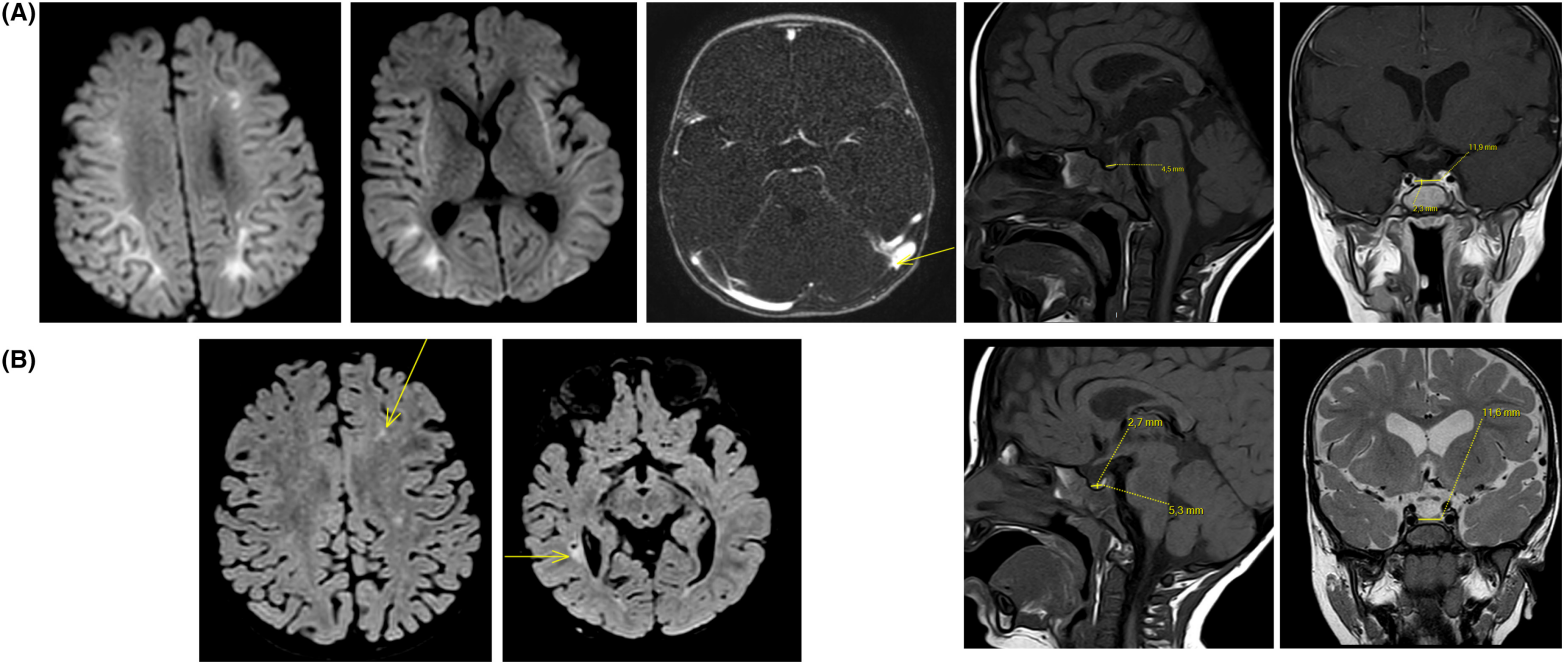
Magnetic resonance imaging performed on Day 2 and at 3 months. (A) MRI performed on Day 2, extensive thrombophlebitis of the superior sagittal sinus and of several cortical veins in the vertex, the torcular, the left transverse sinus and presence of small thrombi in the right transverse sinus. Significant bilateral and symmetrical signal abnormalities of the fronto‐parieto‐temporo‐occipital subcortical white matter and of the external capsules were also visible in diffusion restriction, which seemed rather to be related to metabolic disorders. (B) MRI at 3 months, partial permeabilization of the superior sagittal sinus with persistence of a heterogeneous aspect of the sinus, seat of some linear images probably corresponding to residual filiform thrombi, a complete permeabilization of the transverse sinuses and the torcular and cortical veins, and an appearance of FLAIR signal abnormalities of the periventricular white matter, probably sequelae of the abnormalities visualized in diffusion on the first MRI.
